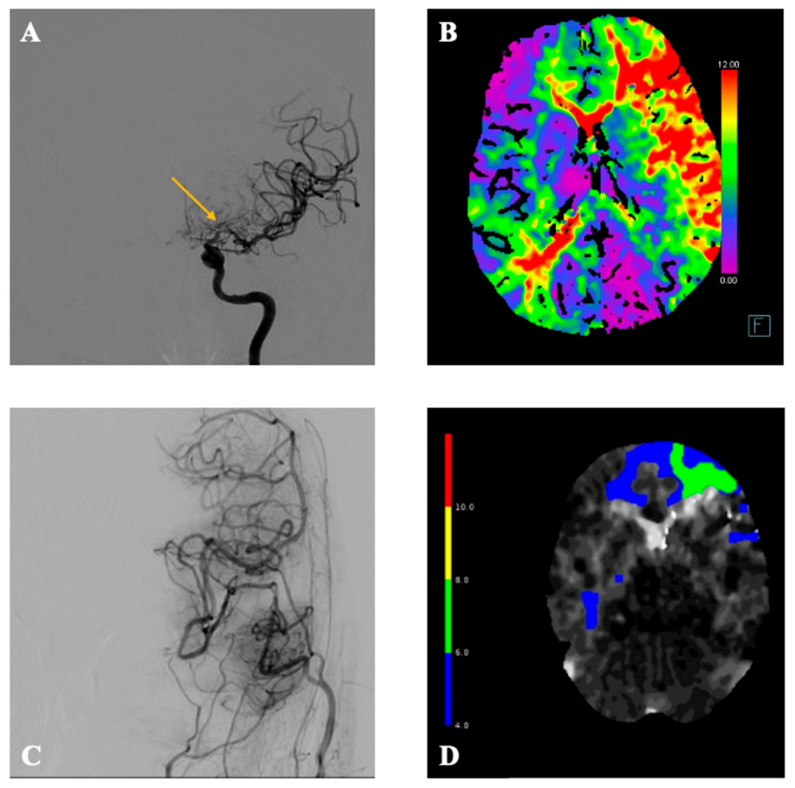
(A,B) show the preoperative digital subtraction angiography and the CT perfusion scan with time-to-maximum scan (Tmax). The yellow arrow in (A) points towards the stenosis of the M1 branch on the left side. Perfusion imaging (B) demonstrates delayed perfusion of the entire left MCA territory with a scale from 4 to 12 s. (C,D) show the imaging studies 2 months postoperatively with the digital subtraction angiography on the left side (C). The postoperative perfusion CT with scale from 4 to 12 s demonstrates largely restored perfusion after the DB STA-MCA bypass with a delayed perfusion frontal left (D).
